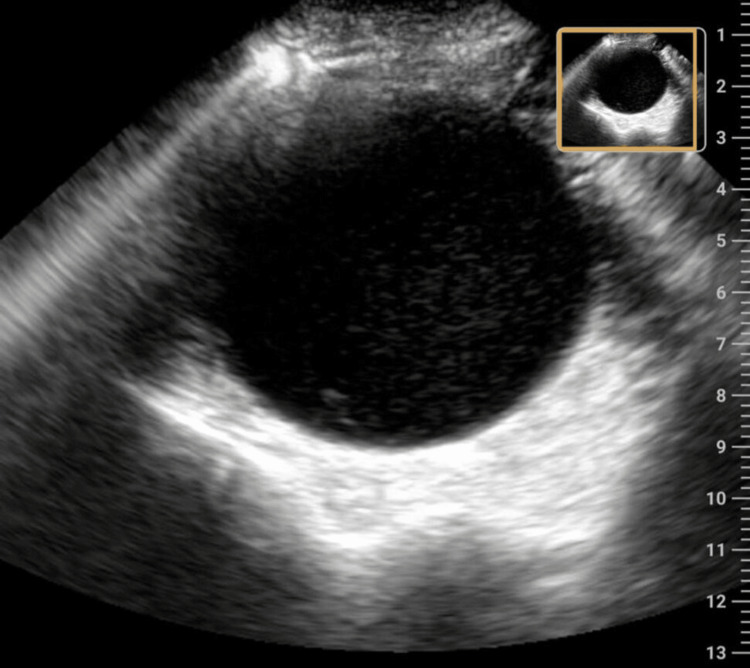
Point-of-care ultrasound demonstrating hematocolpos.
